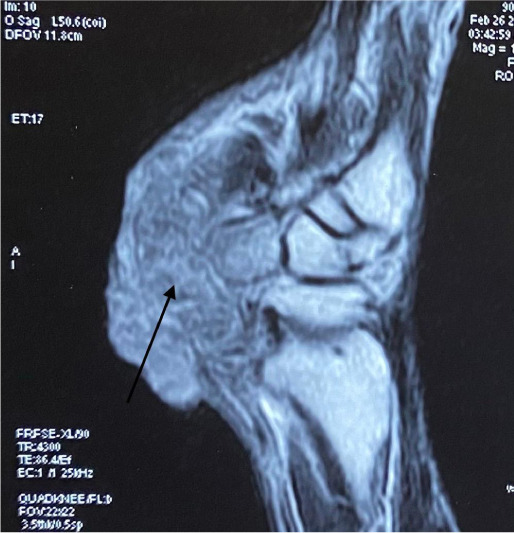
MRI images of the right wrist (T1WI sagittal) showing an ill-defined soft tissue lesion involving the flexor aspect of the distal third of the forearm and wrist (thin black arrow). Lesion involving the pisiform bone (thick black arrow).
